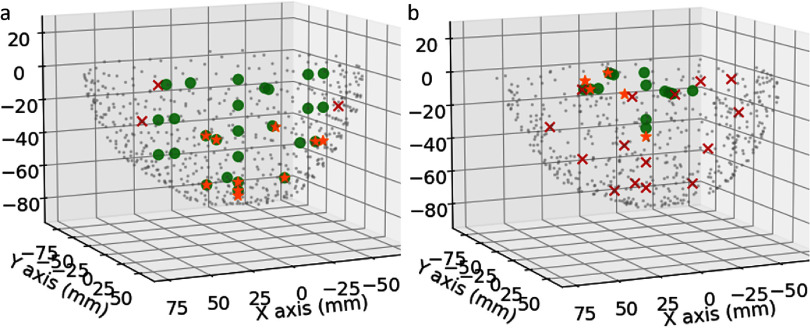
Treatment envelope indicated by ACE detection in skull #1 (a) and skull #2 (b), respectively. Grey dots: vertices on the skull outer surface mesh reconstructed from CT scans. Green dot: on-target cavitation detected; orange star: pre-focal cavitation detected; red X: no cavitation events.
